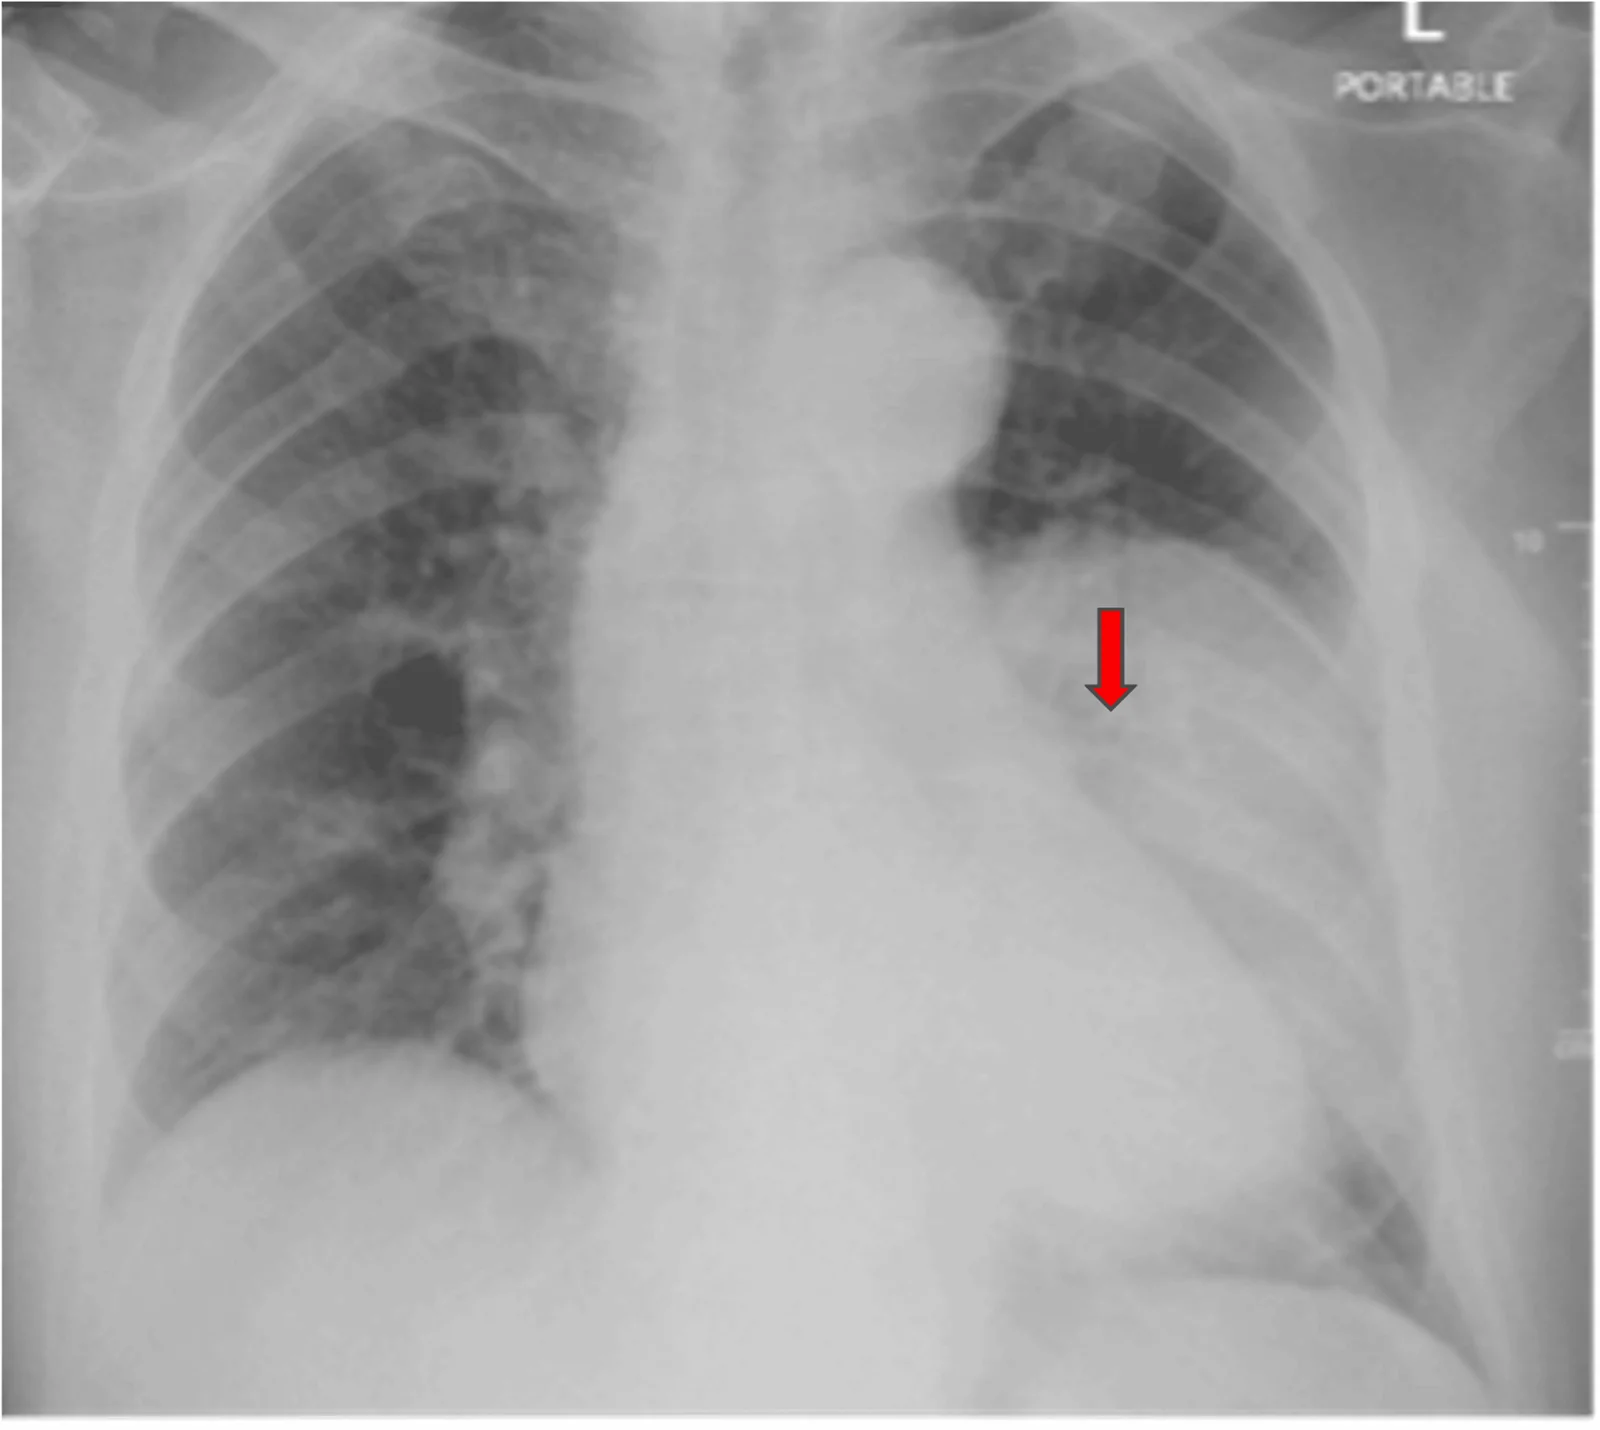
Posteroanterior chest X-ray demonstrating a large ovoid left lung mass measuring approximately 14 cm in craniocaudal diameter consistent with an intrapulmonary soft tissue tumor
